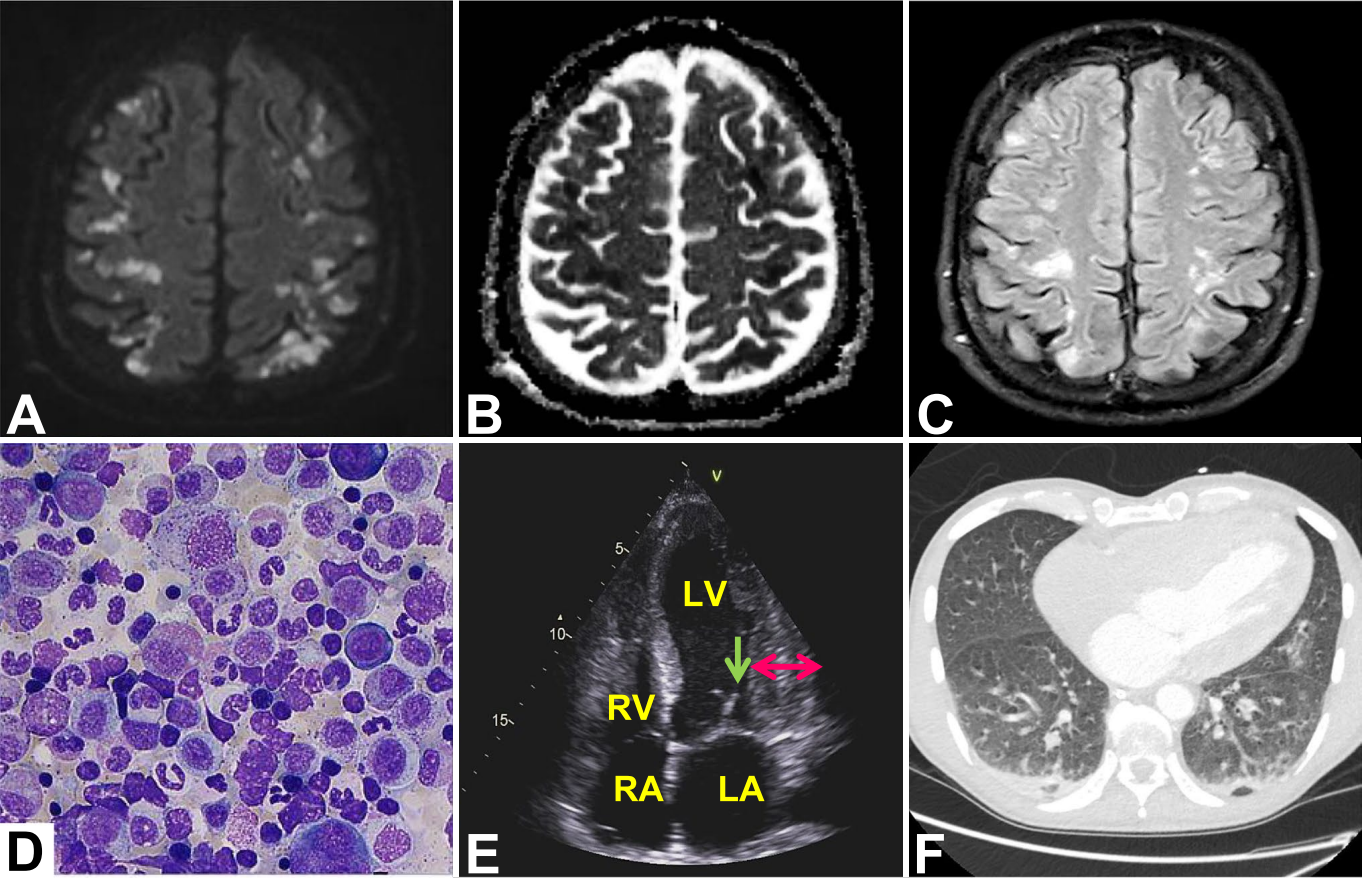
MR imaging demonstrating multiple bilateral restrictions in the diffusion-weighted images in the border zone and the cortex (A and C). Decline in the apparent diffusion coefficient (ADC) (B) corresponding to (A). Fluid attenuated inversion recovery (FLAIR) (C). Bone marrow biopsy revealed hypercellularity with an eosinophilic granulocyte count of 52% and no elevated blast count or fibrosis (D). Transthoracic echocardiogram demonstrated thickened endomyocardium (red arrows) and a floating structure at the mitral valve (green arrow). *LV* left ventricle, *LA* left atrium, *RA* right atrium, and *RV* right ventricle (E). Pulmonary ground glass opacities of interstitial pulmonary fibrosis on CT scan (F)
